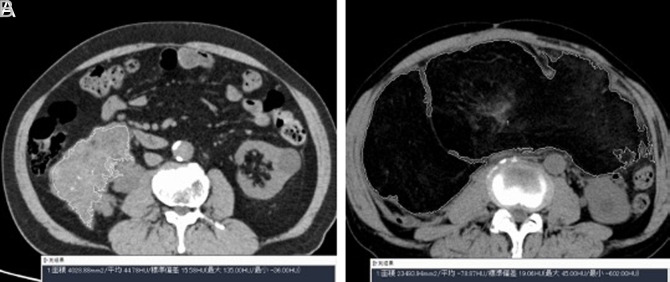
A and B. Representative examples of tumors and their corresponding CT images: A. Cases of dedifferentiated liposarcoma, with a higher HU value of 44.8. B. Case of well-differentiated liposarcoma, with a low HU value of −78.9. CT, computed tomography; HU, Hounsfield unit.
